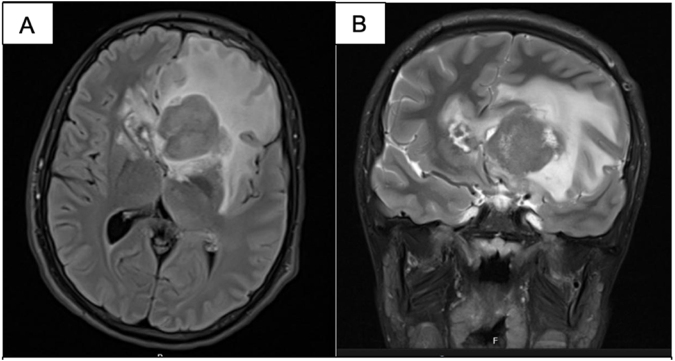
T2/fluid-attenuated inversion recovery (FLAIR) imaging showing subcortical non-enhancing hyperintensity in the frontal lobe extending into the genu of the corpus callosum and into the intervening falx (a, axial; b, coronal).
